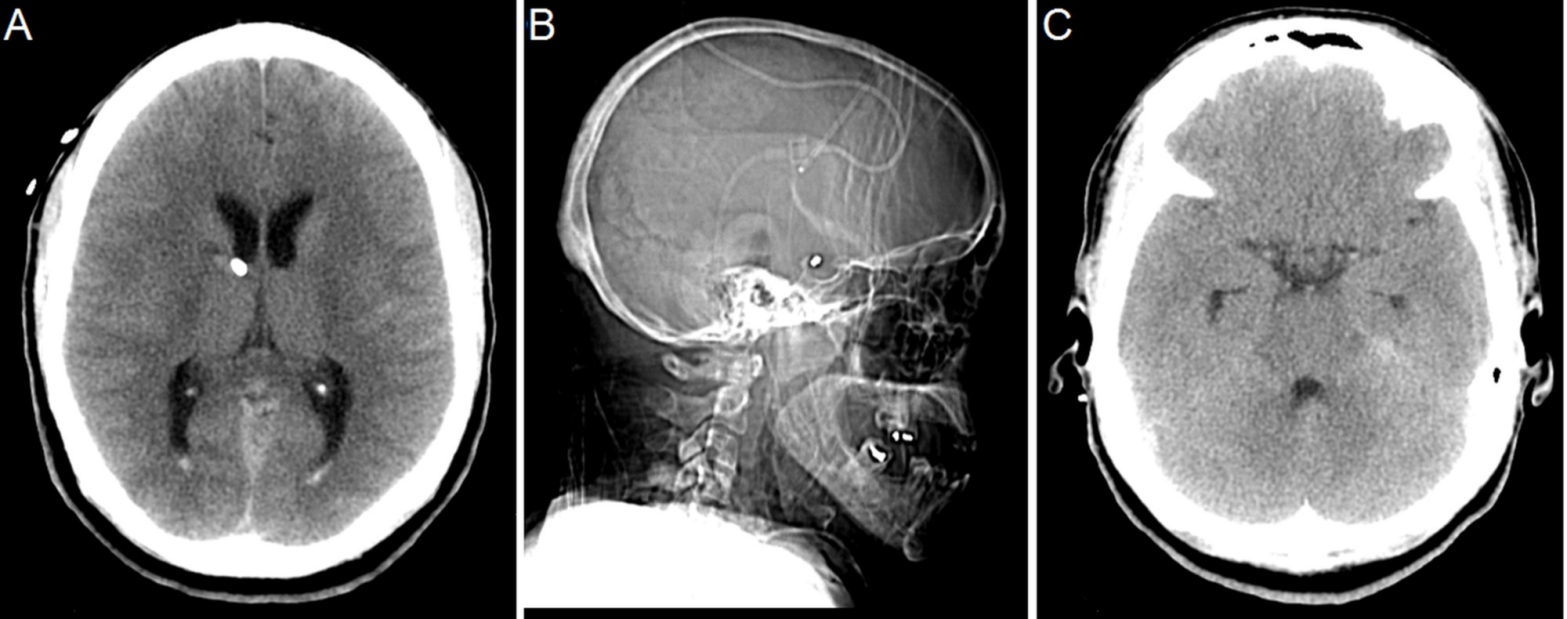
Axial CT head (A) and sagittal X-Ray (B) following adjustment of EVD showing the catheter tip in the Foramen of Monro. Axial CT head (C) showing no tectal blood following external ventricular drains (EVD) adjustment.
